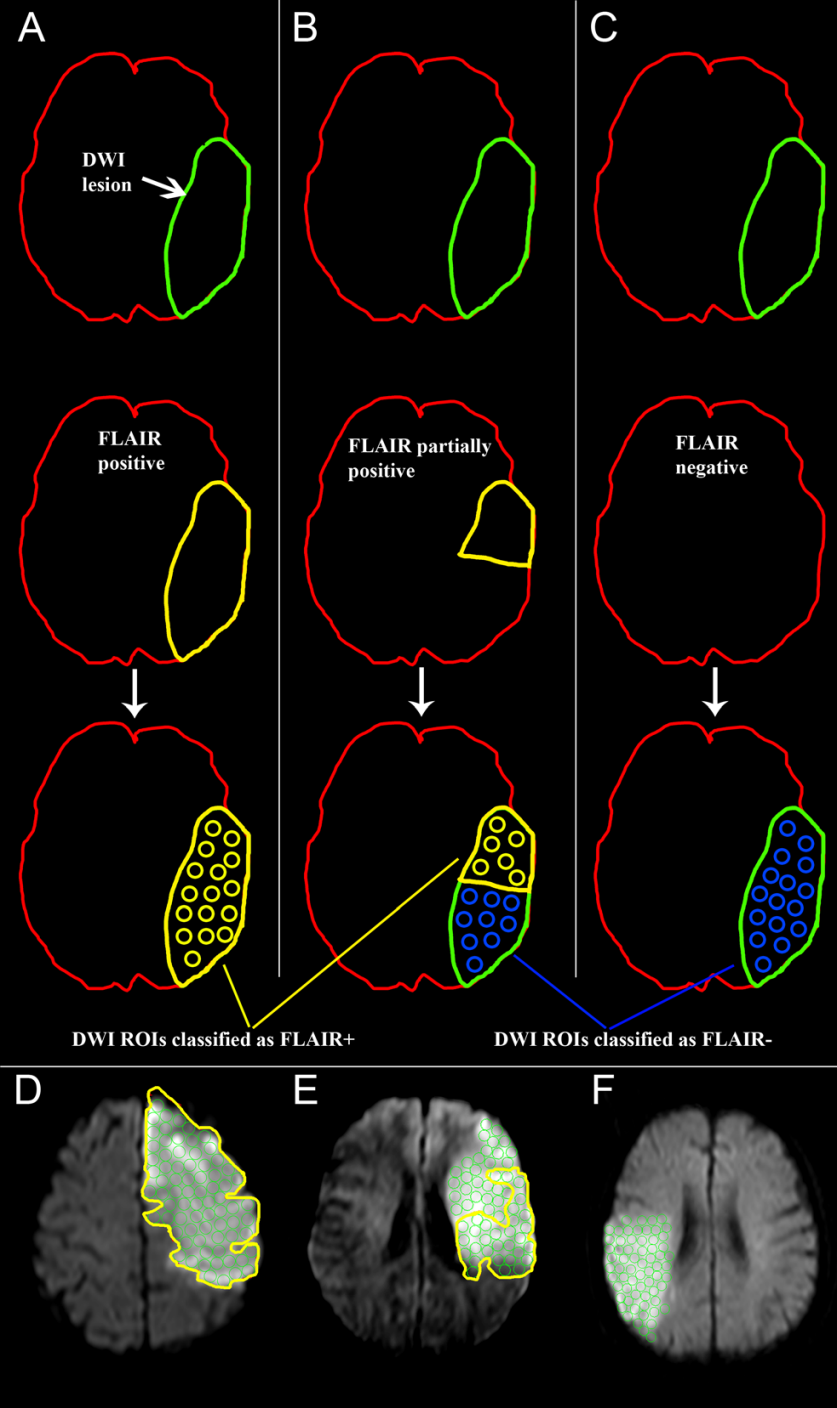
Labeling of lesions in DWI imaging according to the FLAIR pattern. A) In case of a FLAIR lesion encompassing the whole DWI-lesion, the hyperintensity was delineated as a region of interest (ROI) (A, second row). The FLAIR-ROI was then copied on the DWI and filled with 6 mm circular ROIs (A, third row)). These ROIs were classified as FLAIR+. B) In cases, in which the FLAIR-ROI did not completely match the DWI-lesion (B, second row), ROIs inside the FLAIR-ROI were classified as FLAIR+, and those outside as FLAIR- (B, third row). C) If no FLAIR lesion was identified (C, second row), the whole DWI lesion was filled with circular ROIs, which were classified as FLAIR- (C, third row). These steps were performed equally in ADC-maps. D), E) and F) show examples in analogy to the scheme, D) showing a patient, where the delineated FLAIR-ROI encompasses the whole DWI-lesion, E) depicting a patient, where the FLAIR-lesion only partially covers the DWI-lesion. Lastly, F) shows a patient, where all DWI-ROIs were labelled as FLAIR- in the absence of a visible FLAIR lesion.
